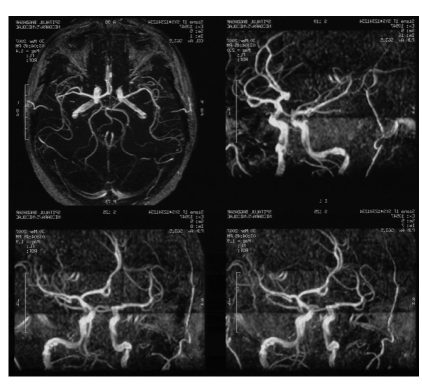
Angio-MRI two years post gamma-knife treatment.
